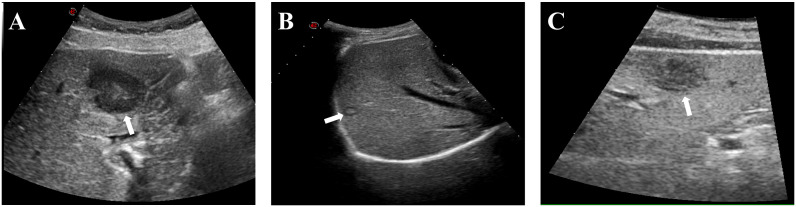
Typical ultrasound images of patients with liver tumors at difficult locations. (A) Liver tumor adjacent to the gastrointestinal tract (white arrow); (B) Liver tumor adjacent to the diaphragm (white arrow); (C) Liver subcapsular tumor (white arrow).
